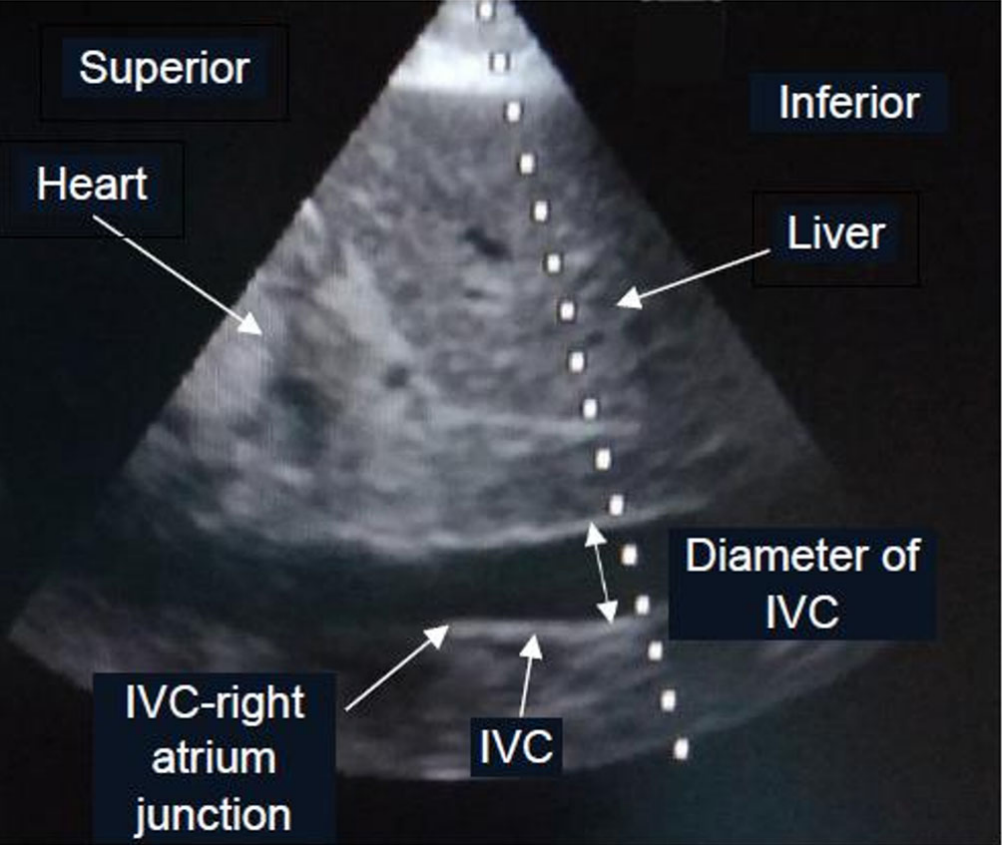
Ultrasonographic findings of IVC diameter measurement
